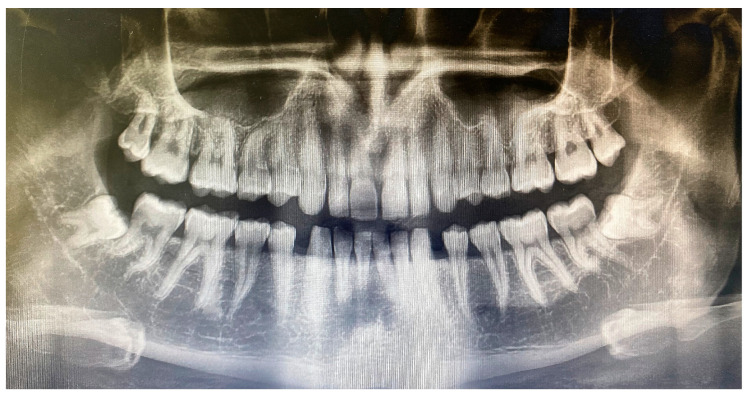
Panoramic radiograph of a patient with a similarly positioned, symmetrical, and bilaterally impacted lower third molar tooth.
